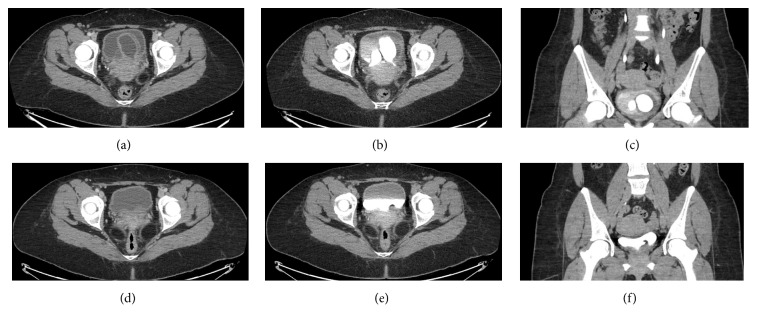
Preoperative (a, b, c) and postoperative (d, e, f) comparison of computed tomography (CT) scans. Preoperative triphasic CT scan images. (a) Contrast-infused axial images of the pelvis demonstrating two large bilateral intravesical cavities. (b) Axial delayed-phase images of the pelvis confirming the diagnosis of bilateral ureteroceles. (c) Coronal delayed-phase images of the pelvis provide another view of the bilateral ureteroceles. Postoperative triphasic CT scan images. (d) Contrast-infused axial images of the pelvis demonstrating the absence of intravesical cavities. (e) Axial delayed-phase images of the pelvis confirming the absence of any residual ureteroceles. (f) Coronal delayed-phase images of the pelvis.
